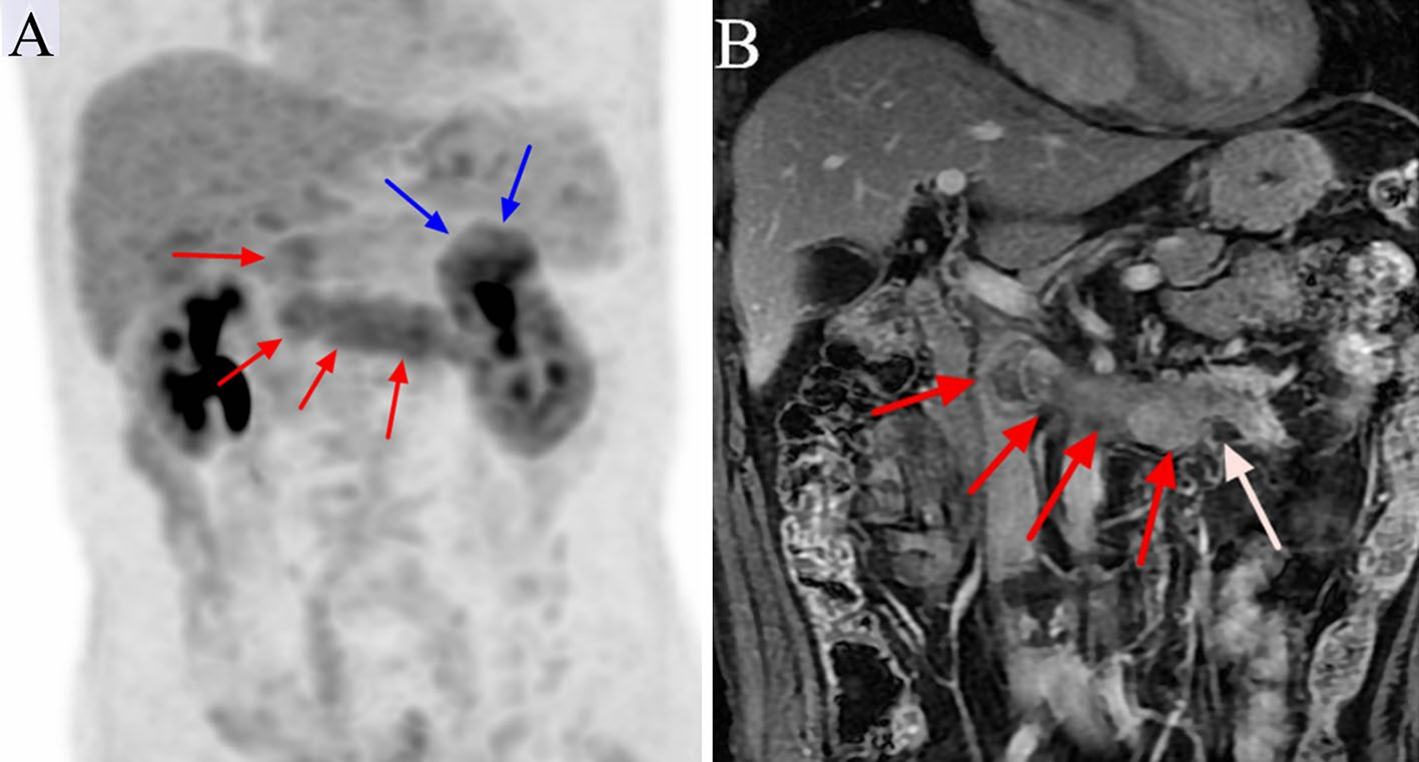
^18^F-FDG PET/CT and contrast-enhanced MRI (CEMRI) scans of a 75-year-old man with clear cell carcinoma of the left kidney associated with a level II venous tumour thrombus (VTT) and small venous bland thrombus (VBT). The VTT did not invade the venous wall and was graded as level II by PET/CT and CEMRI. The site of focally increased FDG uptake (SUVmax 5.8) on the PET maximum intensity projection image is the primary tumour (blue arrow) (a). A PET maximum intensity projection image (a) shows a site of pathologically increased FDG uptake (SUVmax 4.4) from the left renal vein to the inferior vena cava that is the VTT (red arrow). The VTT has a regular shape, is homogeneously enhanced, and completely occludes the inferior vena cava lumen on coronal CEMRI (red arrow) (b). The small VBT shows no enhancement on coronal CEMRI (white arrow) (b).
